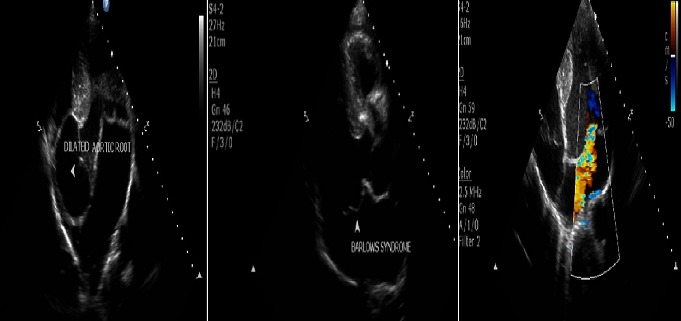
(A) echocardiographic findings of a dilated aortic root; (B) prolapsing mitral valve leaflet; (C) mitral valve regurgitation
